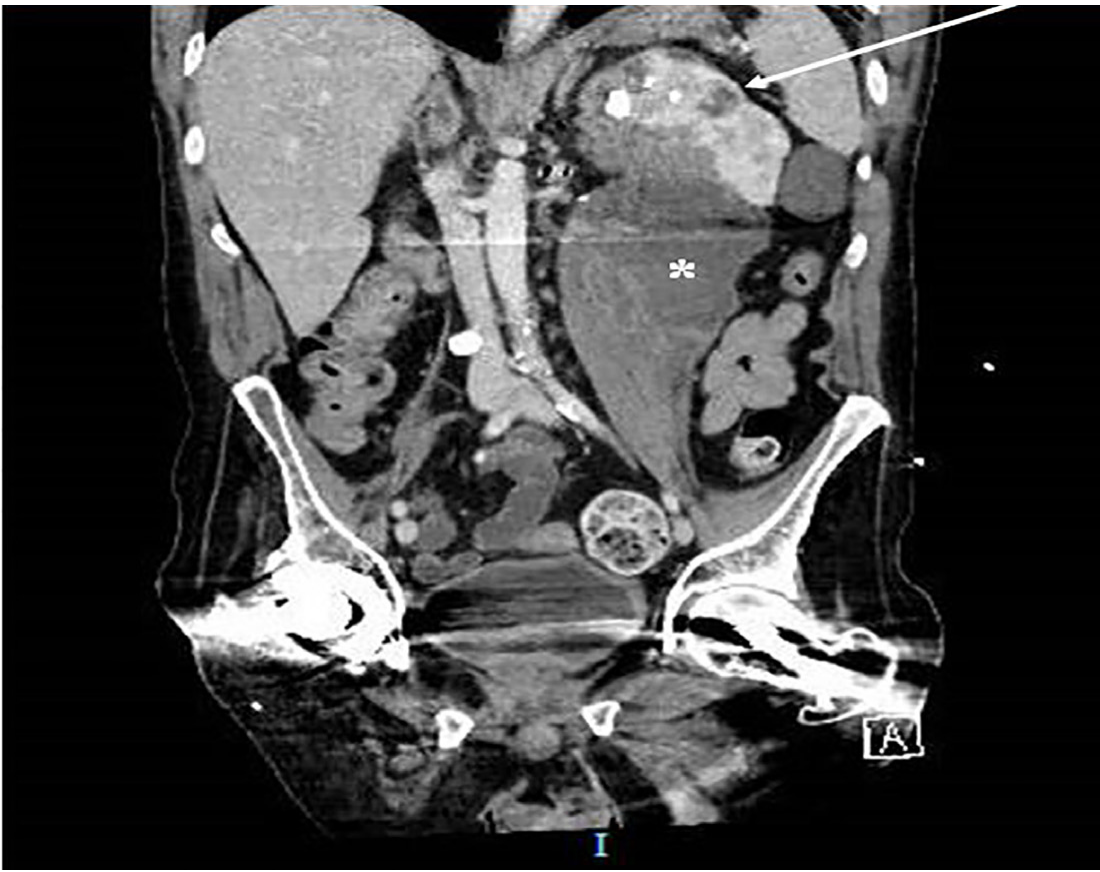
Computed tomography, coronal image, demonstrating large fluid collection (*) centered over the left psoas. The left kidney is displaced and demonstrates a staghorn calculus with cystic changes contiguous with the fluid collection (arrow).
